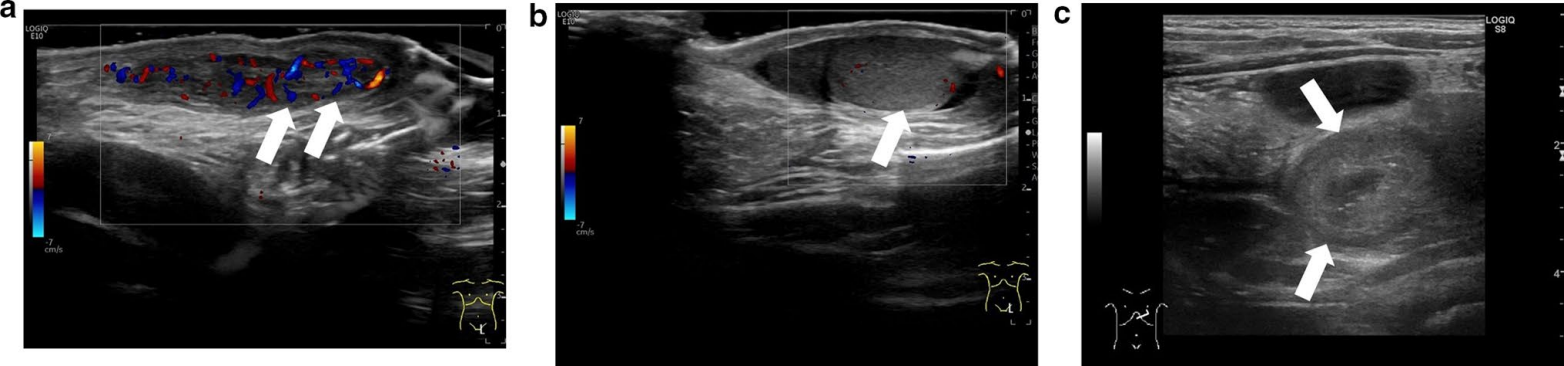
Ultrasonographic images of a 4-year-old with left acute scrotum with IgA vasculitis. a Swelling of the left epididymis and increased vascularity are evident. b Vascular flow within the left testis is preserved. c Wall thickening and edematous changes are evident in the dermis of the descending duodenum of the right and left scrota. Purpura was detected, leading to the diagnosis of IgA vasculitis
